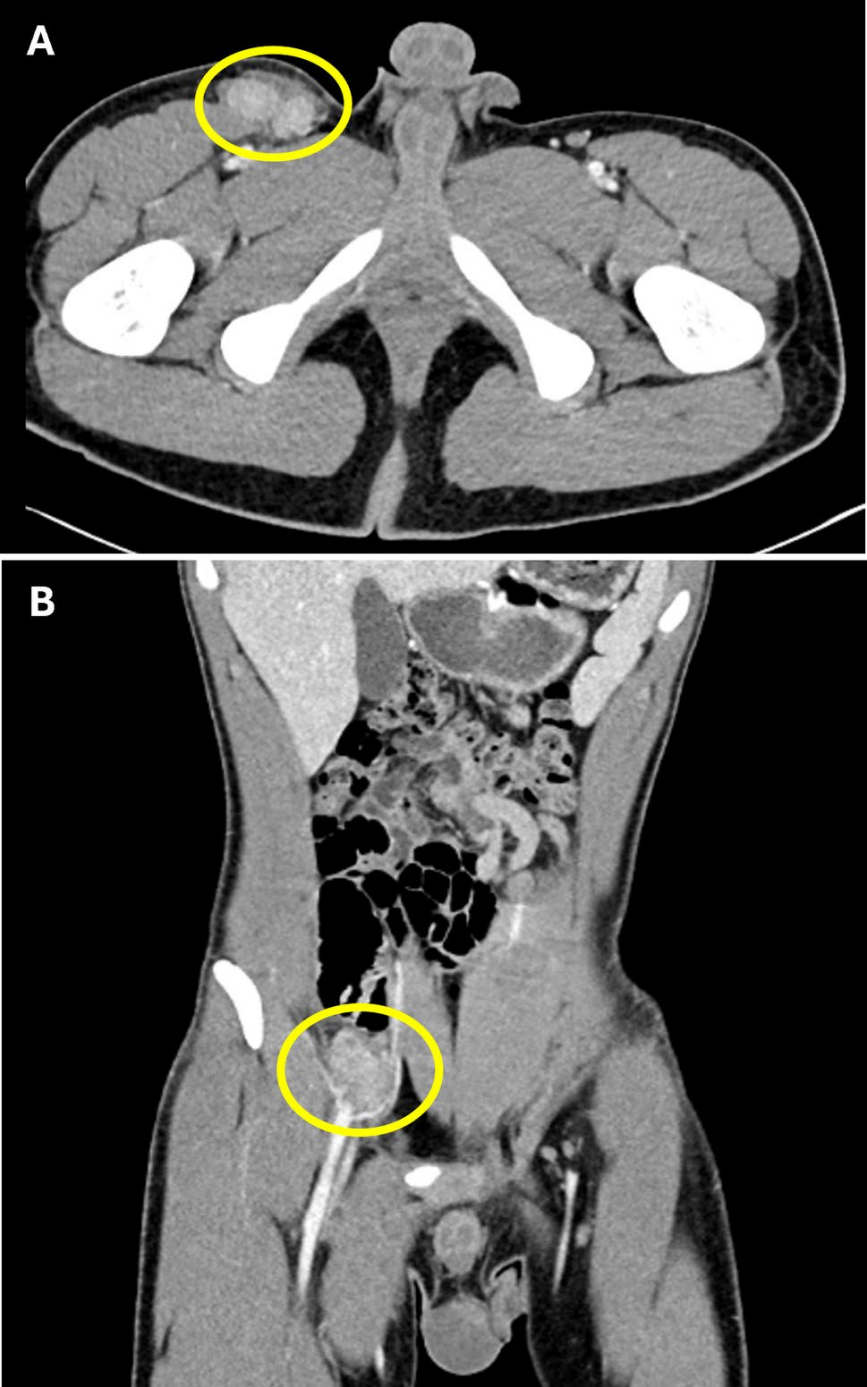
Multifocal lymphadenopathy on computed tomography (CT) scan. Axial (A) and coronal (B) CT scan images show enhancing enlarged lymph nodes in the inguinal (A) and external iliac (B) regions, highlighted by yellow circles
